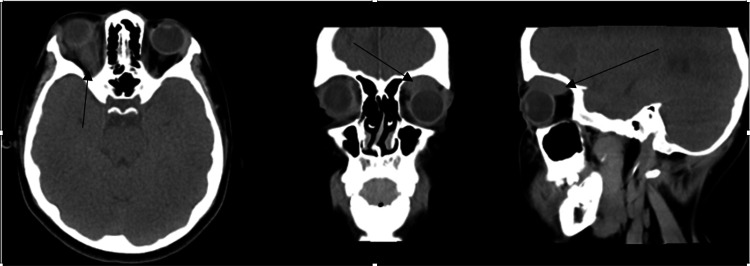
Axial, coronal, and sagittal views of CT scan image showing fullness in the right superior rectus muscle, likely hematoma (black arrow).
